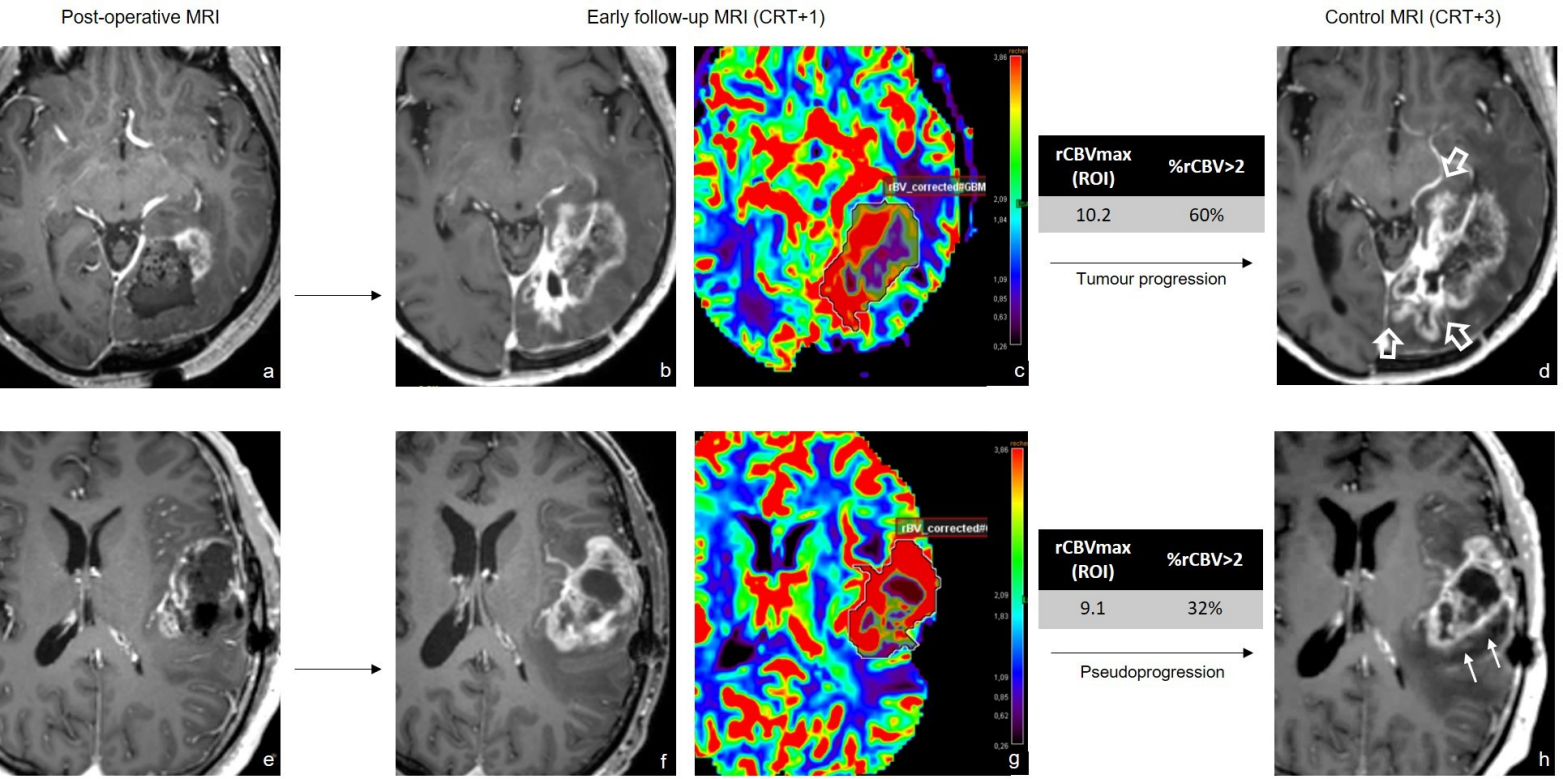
Longitudinal follow-up of two different glioblastomas with similar high rCBVmax (ROI) and different %rCBV>2. Axial contrast-enhanced T1-weighted images (CE-T1WI) of post-operative MRI (a and e), showing progressive lesions on CE-T1WI of CRT+1 MRI (b and f) with their corrected rCBV maps (c and g) and subsequent evolution on CRT+3 MRI (d and h). Top line (a-d) shows tumour progression (arrows) at CRT+3, calculated rCBVmax was 10.1 and %rCBV>2 was 60%. Bottom line (d-f) demonstrates regression of the lesion (thin arrows) indicative of pseudoprogression, despite high rCBVmax (9.1), %rCBV>2 was 32% and could have predicted pseudoprogression.
